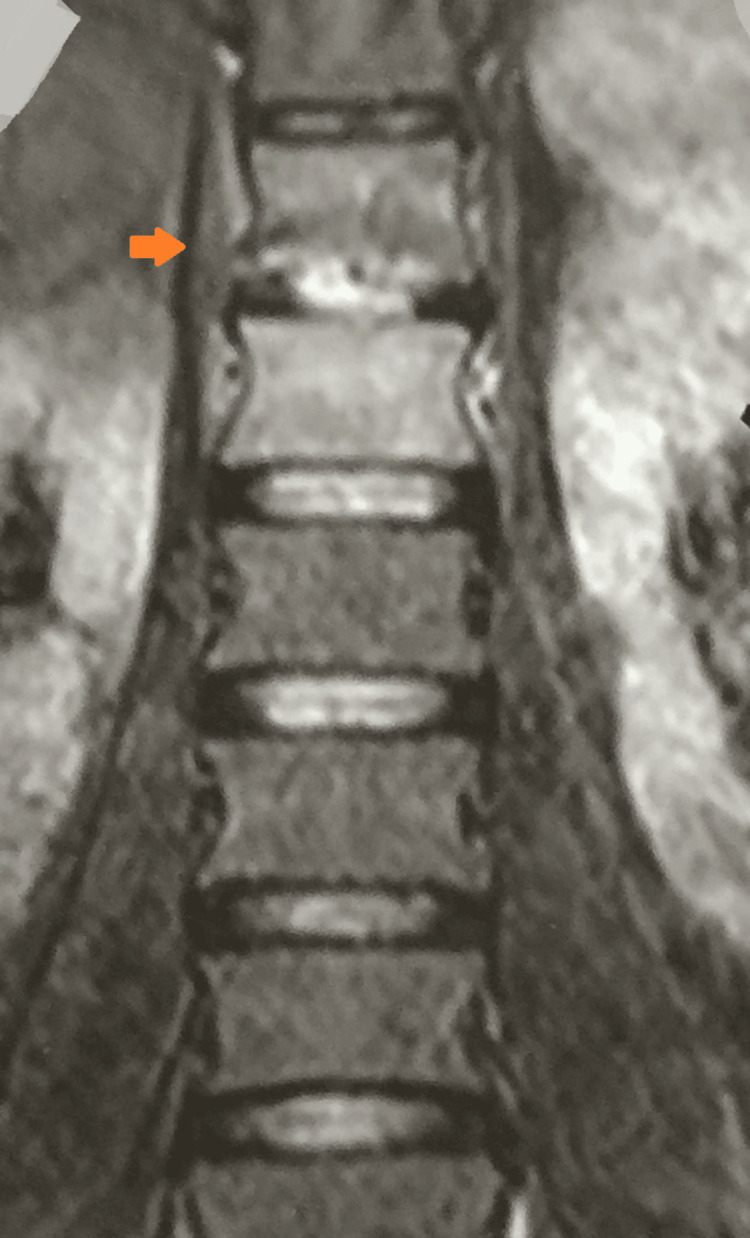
MRI of the dorso-lumbar spine showing bone marrow edema at D12 and L1 vertebral bodies with cortical destruction of the posterior part of D12 vertebral
